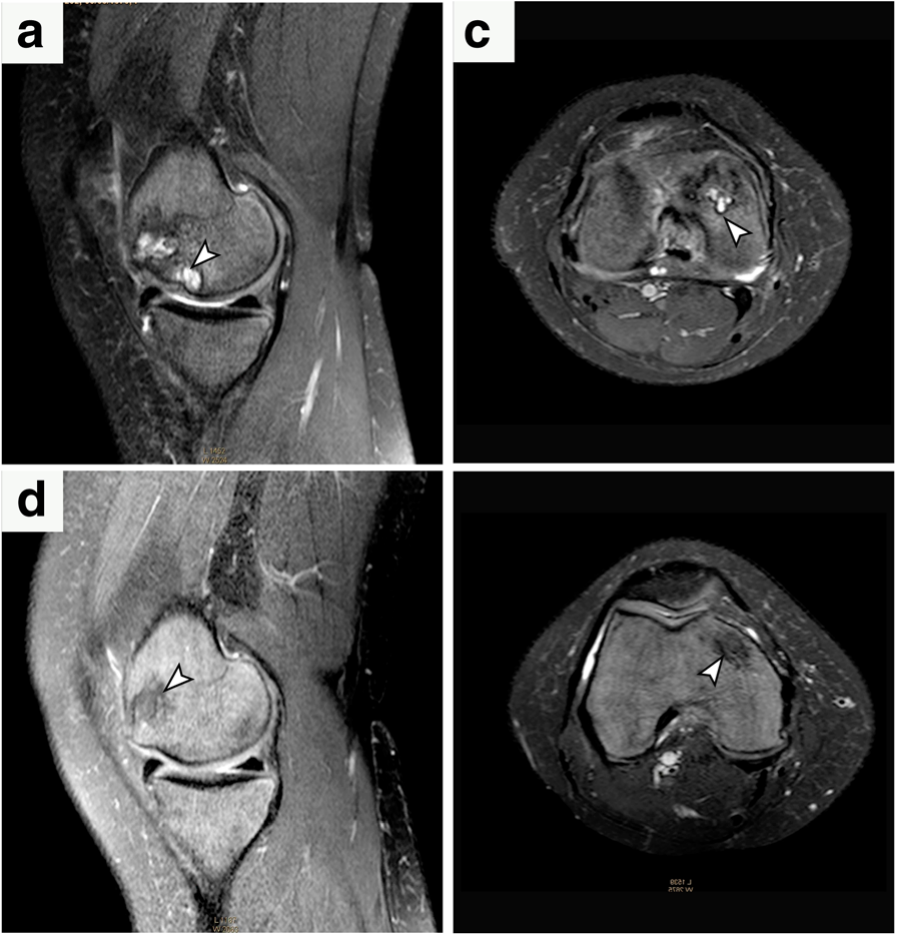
Bilateral osteonecrosis of the knees in a symptomatic 39-year-old woman with SCD. (a - d) Proton density fat-suppressed (a, c – sagittal) and T2-weighted (b, d - axial) sequences demonstrate subchondral abnormalities (arrowheads) located in the medial femoral condyle (weight bearing zones). Subcortical high-intensity inner line (a – c) representing granulation tissue. MRI scans before surgery shows osteonecrotic lesion in Ficat I and II on left and right knees, respectively. Both knees were treated with BMC implantation
